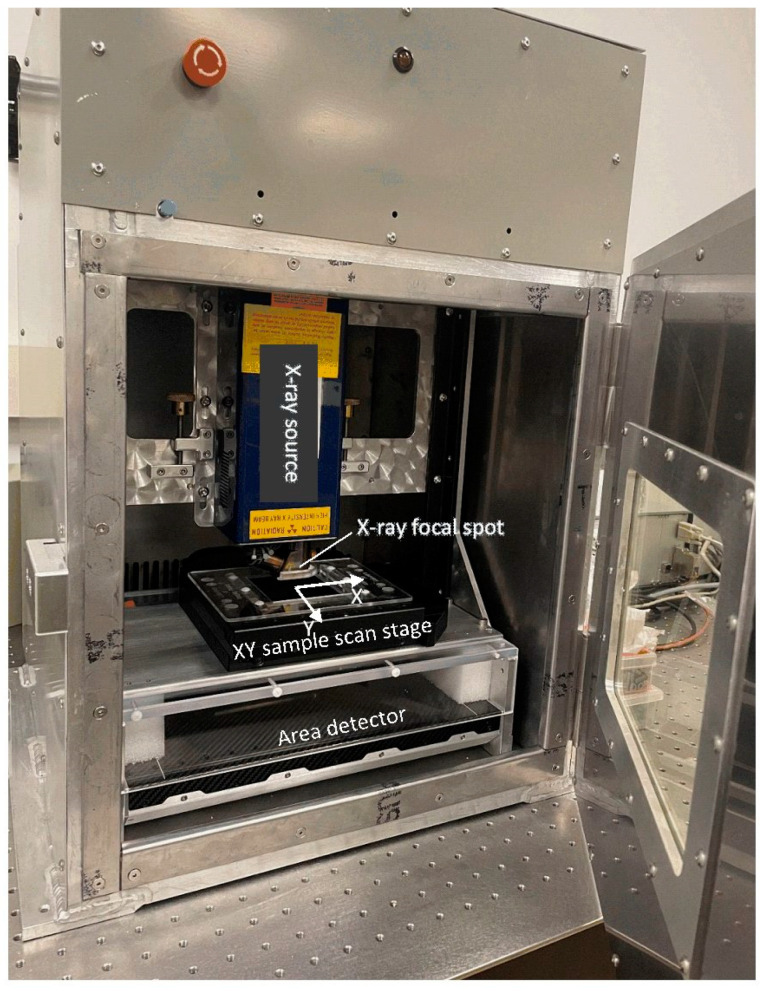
The X-ray micro tomosynthesis scanner with the radiation enclosure. The outer dimensions of the enclosure are 45 × 52 × 66 cm (width × depth × height). The motorized sample stage moves nominally in the horizontal plane. The sample is scanned horizontally across the vertical cone beam in either x or y direction. Flat samples are usually scanned in a horizontal plane near the X-ray focal spot to maximize the photon flux density through the sample and the geometric magnification for greater contrast-to-noise ration and resolution.
